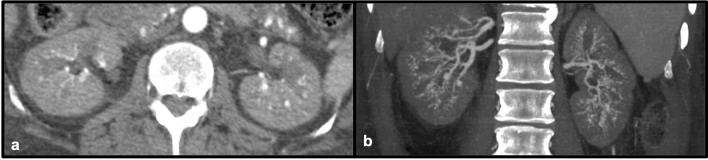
49-year-old male with multiple medical problems found to have laboratory evidence of drug-induced lupus. Axial (a) and coronal maximum-intensity projection (MIP) contrast-enhanced CT images (b) demonstrate innumerable aneurysms of varying sizes involving several renal artery branches bilaterally. This patient expired 9 days after this CT scan
